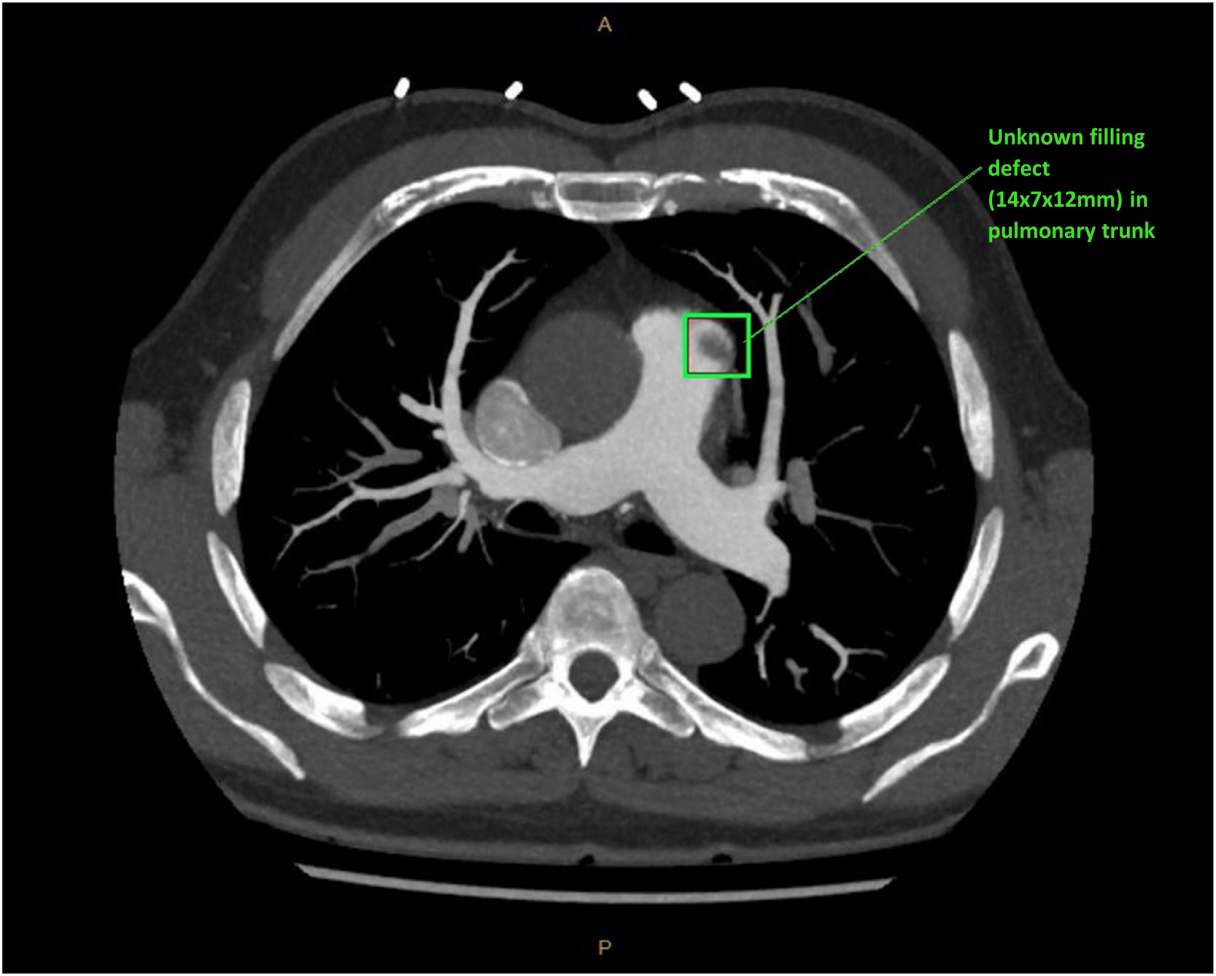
CTPA depicts an unknown filling defect in the pulmonary trunk region. CTPA, CT pulmonary angiogram.
